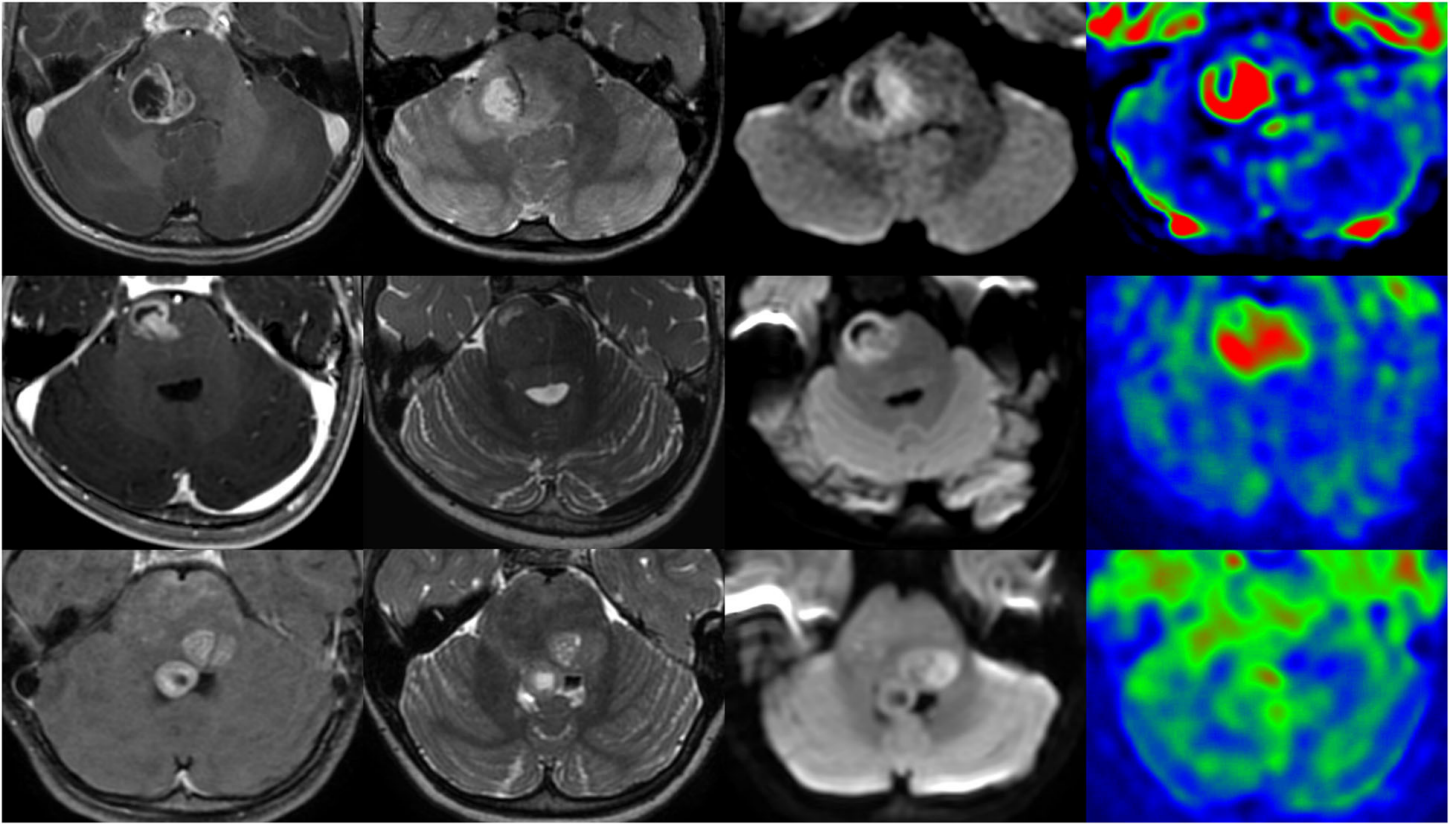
Radiological features of three pontine HGG-MYCN. Each line represents representative images of each patient (First line: Case 1; second line: Case 3; third line: Case 4). First row shows T1-weighted images after contrast media injection, second row T2-weighted images, third row diffusion-weighted images, and fourth row cerebral blood volume map using arterial spin labeling. All tumors display necrotic part with intense annular enhancement and surrounding T2-weighted hyperintense signal. Diffusion is restricted in the solid part of the tumors. Cerebral blood flow is high using arterial spin labeling
